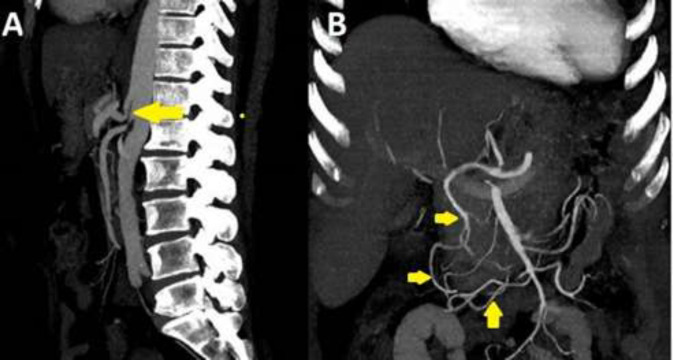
Computed tomography angiography shows the characteristic hook appearance of the proximal celiac artery and focal narrowing with poststenotic dilation. A: Significant stenosis is also observed in the superior mesenteric artery root. B: Significant collateral flow is observed between the celiac artery and the superior mesenteric artery through the gastroduodenal artery
